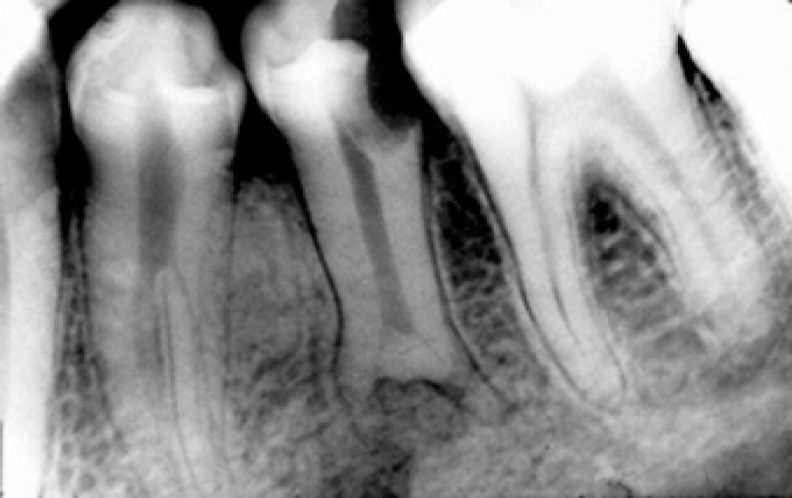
preoperative diagnostic radiograph
